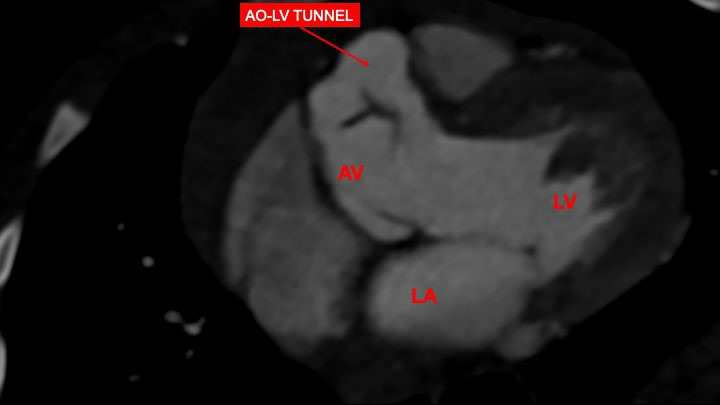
Contrast-enhanced computed tomography. This scan is
